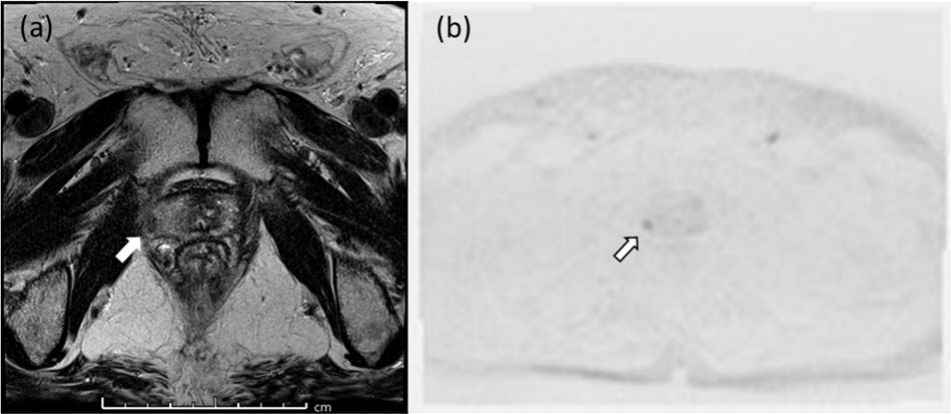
Pelvic magnetic resonance imaging revealed a lesion in the right peripheral zone of the prostate (a, b).
